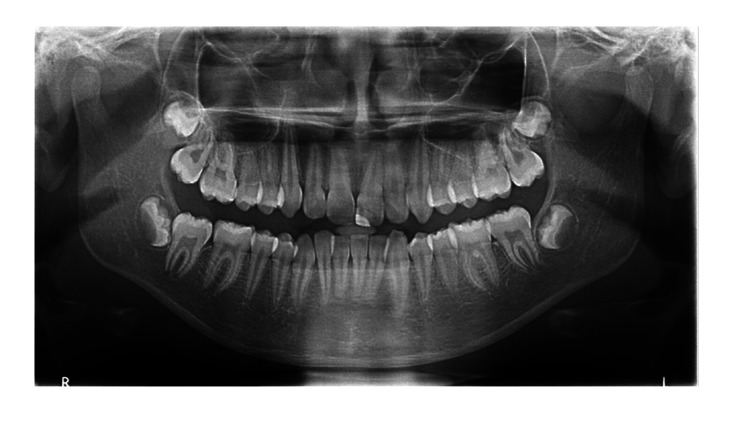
Ideal digital orthopantomogram.
